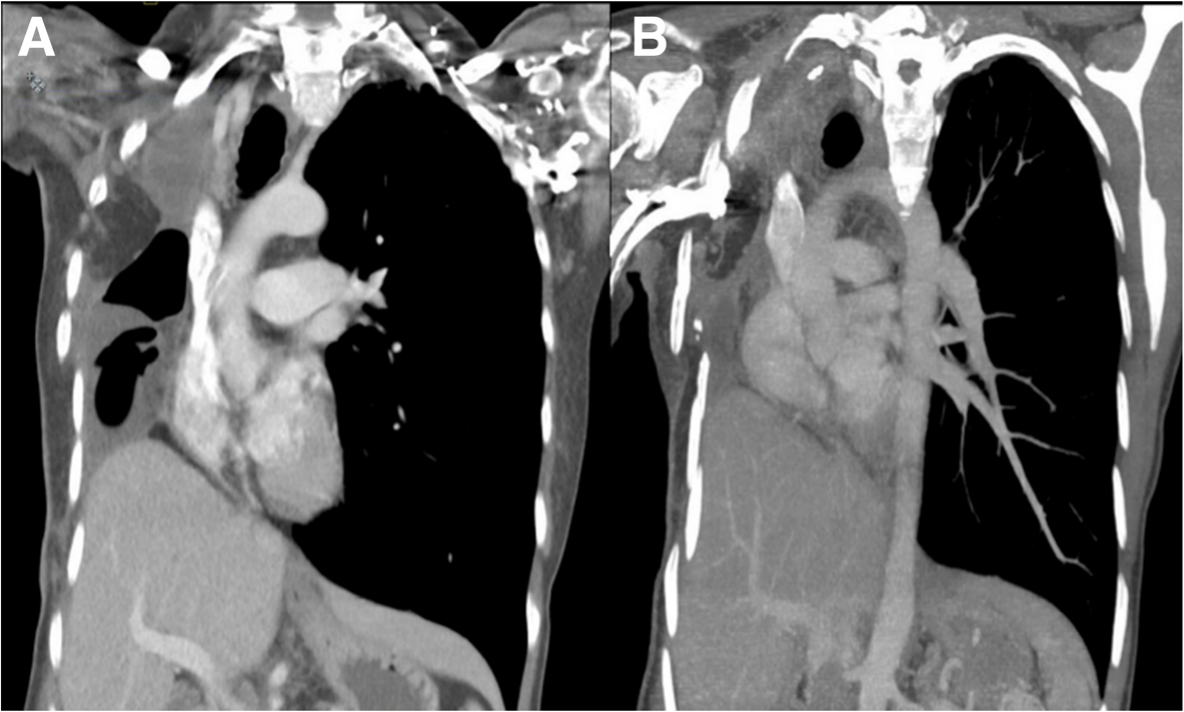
CT scans prior to postpneumonectomy syndrome correction: a initially showing multiloculated air-fluid levels three months after right pneumonectomy shortly after closure of a bronchopleural fistula, and b progressive rightward mediastinal shift with obliteration and resolution of the loculations
